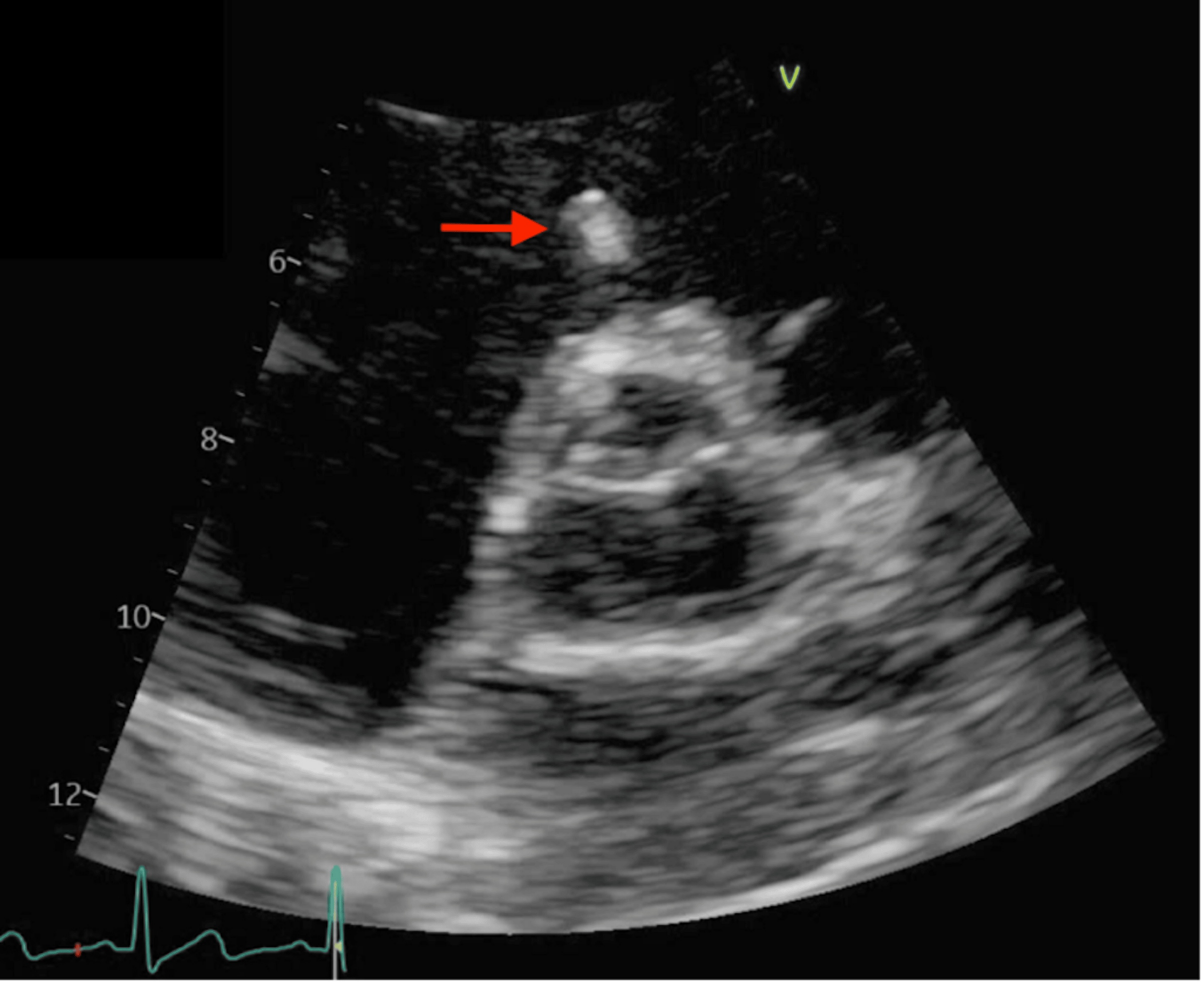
Transesophageal echocardiogram displaying a hyperechoic mobile mass (shown with a red arrow), suggestive of cardiac Kaposi sarcoma.
